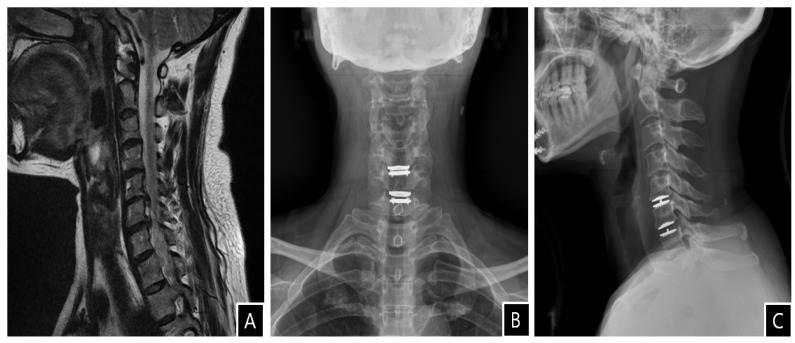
(A) T2-weighted sagittal magnetic resonance image reveals foraminal stenosis at the C5–C6 and C6–C7 levels. (B,C) Postoperative simple radiographs show cervical disc arthroplasty implants at the C5–C6 and C6–C7 levels. A 51-year-old woman with a medical history of hypertension presented with a 6-month history of progressive radiculopathy of the left side that had not responded to conservative treatment. Magnetic resonance imaging (MRI) revealed foraminal stenosis and focal cord compression at the C5–C6 and C6–C7 levels (Figure 1A). She was offered and consented to surgery in the form of anterior discectomy and ADR. The routine laboratory test results of the blood and electrolytes were within the normal ranges. Anesthetic preoperative assessment 2 weeks prior to surgery also showed no specific findings. She underwent elective C5–C6 and C6–C7 anterior cervical decompression and ADR using Mobi-C prostheses (LDR Spine, Zimmer) (Figure 1B,C).
